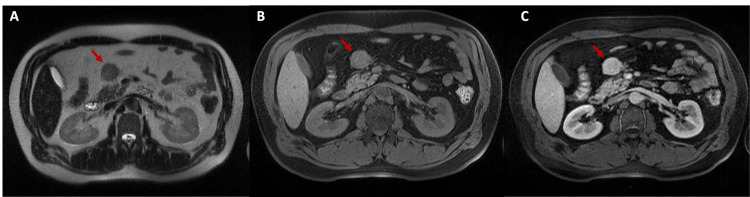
MRI scanning results. A) MRI T2 HASTE axial image showing a T2 hypointense mesenteric mass. B) MRI T1 fat-saturated pre-contrast axial image showing a T1 isointense mesenteric mass, indicating an absence of a fat component. C) MRI T1 fat-saturated post-contrast axial image showing enhancement in the mesenteric mass, suggesting hypervascularity.
